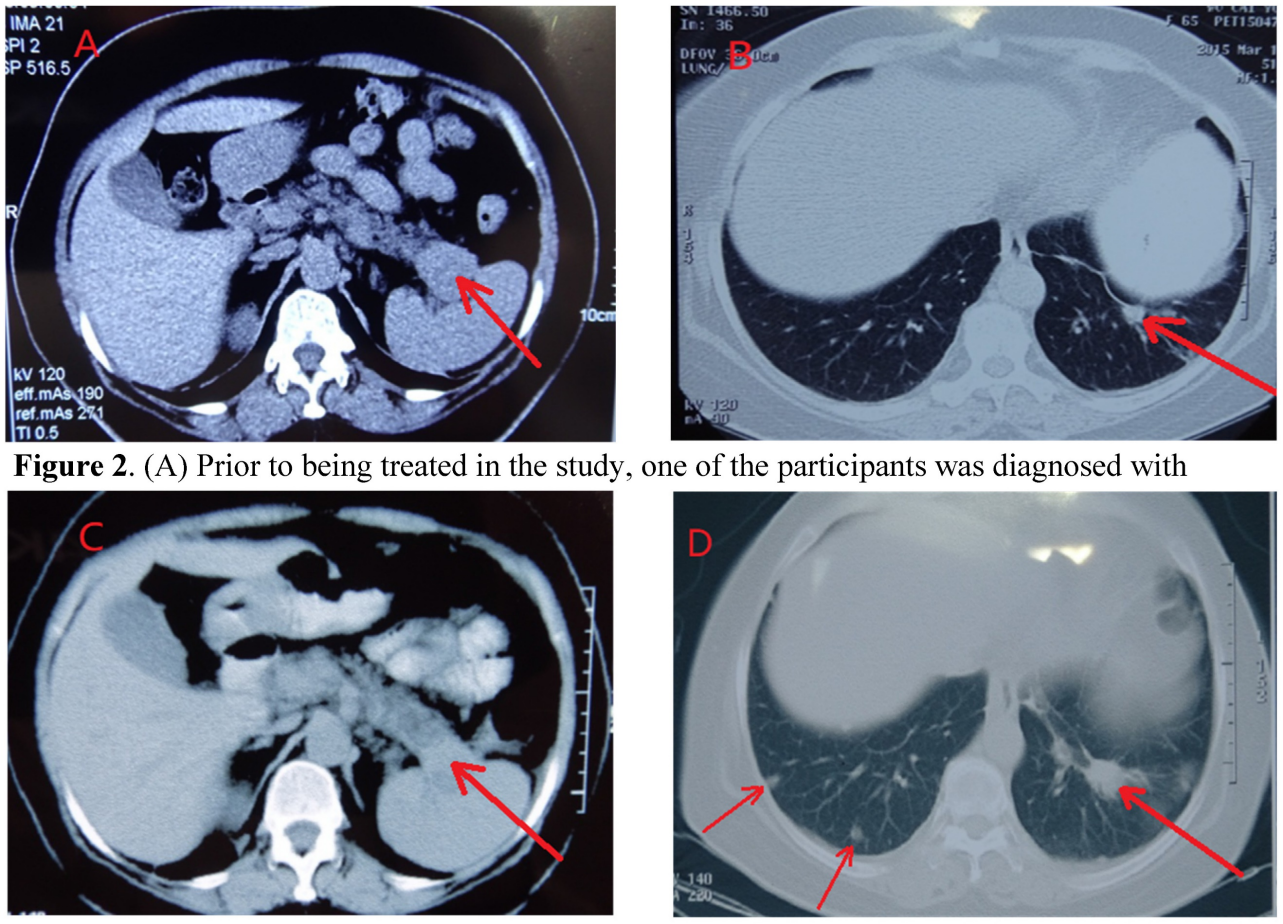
CT of Control of Metastasis in the Lung through Systemic Immunogenicity of TAA. (A) Prior to being treated in the study, one of the participants was diagnosed with pancreatic cancer, originating from the tail of the pancreatic organ. The tumor is at the backside of the pancreas (marked by arrow). (B) Pre-study treatment, the participant's left lung metastasized. The arrow signifies the small mass (marked by arrow) on the scan qualifies the clinical diagnosis of metastasis, without a CT or pathological examination. (C) Two months after HECDI treatment, the pancreatic tumor contracted and was subsequently stable for 2 years. (D) After two years, metastasis originating from the left lung was recognized because the mass on the participant's left lung, initially developed two years ago, presented as more substantial than the newer mass seen on the participant's right lung (right arrow). The arrows on the left indicated new metastasis in left that was not present two years ago.
